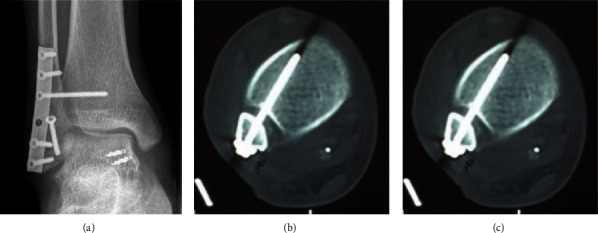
Postoperative imaging. (a) X-ray showed a commendable reduction and fixation. (b) CT demonstrated the position of the distal tibiofibular joint screw was good. (c) CT showed the reduction and alignment were satisfactory.
